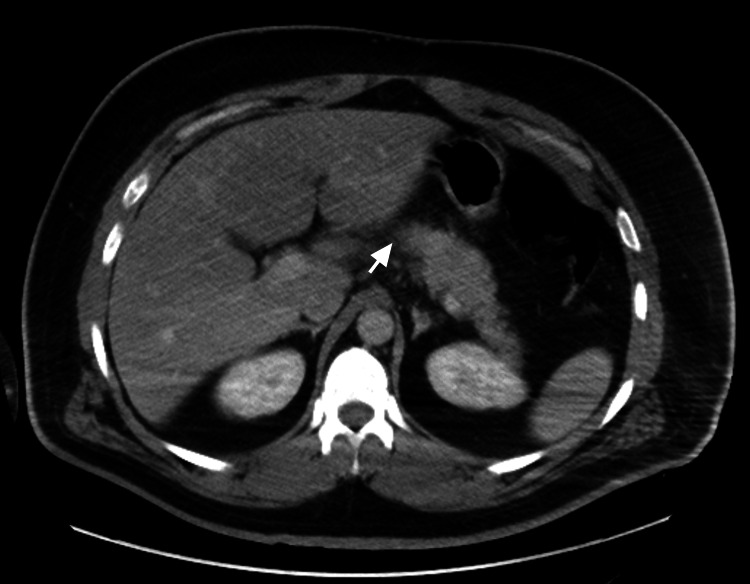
Computed tomography scan without contrast of abdomen. CT scan of the abdomen without contrast showing peripancreatic fat stranding (white arrow), findings consistent with acute pancreatitis.
